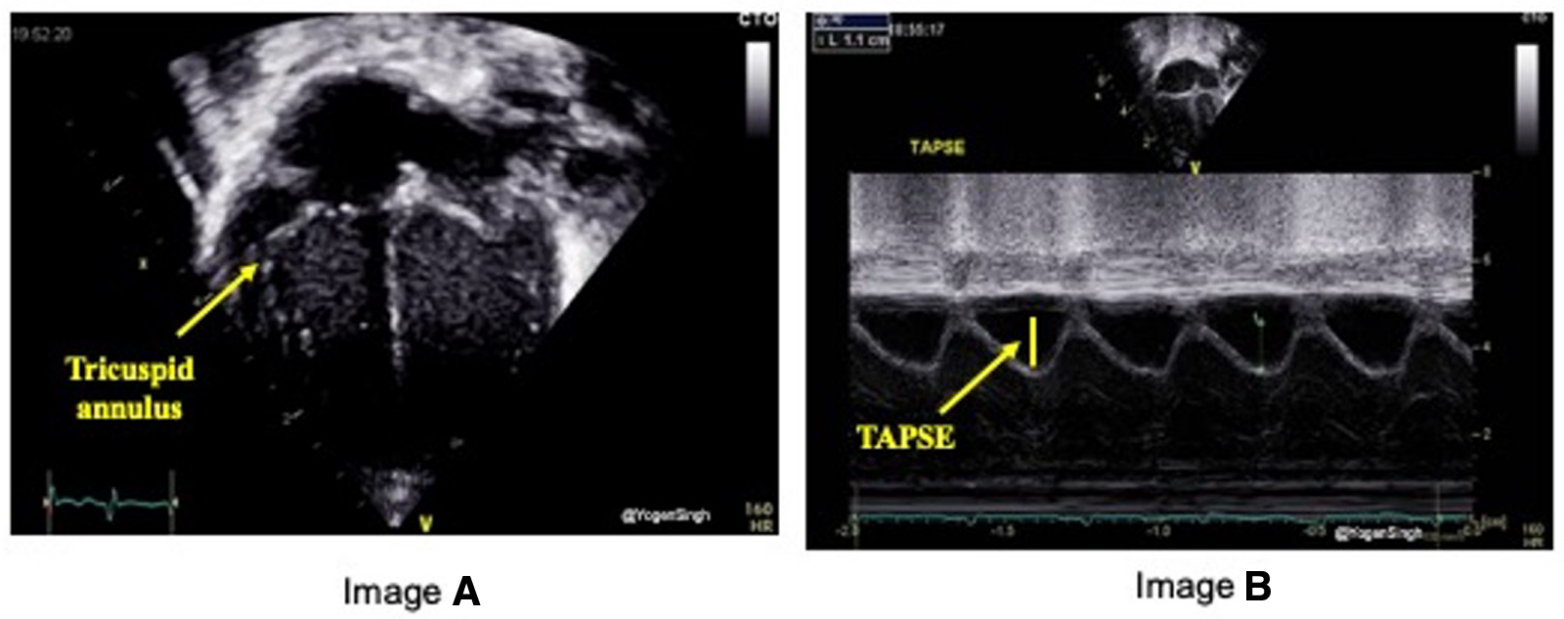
Tricuspid annular plane systolic excursion (TAPSE) measurement on echocardiography. TAPSE reflects the longitudinal movement of the tricuspid annulus towards apex in systole measured on M-mode (image “B”) in Apical 4-chamber view (image “A”).
